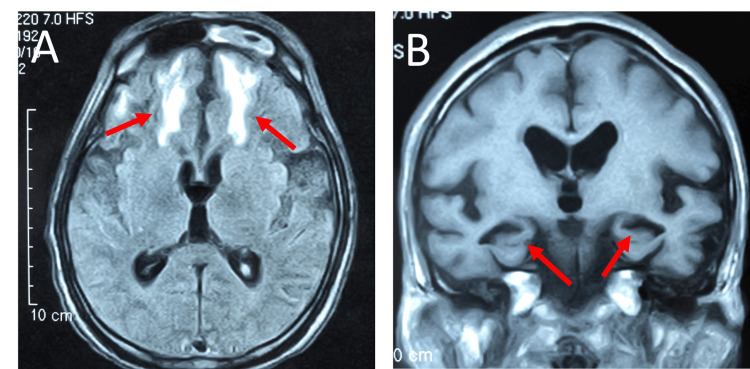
Brain magnetic resonance imaging of patient 2 (A) Fluid-attenuated inversion recovery (FLAIR) image shows hyperintensities in the periventricular white matter around the anterior horn of the lateral ventricles (arrows). (B) T1-weighted coronal image shows mesial temporal lobe atrophy (arrows).
